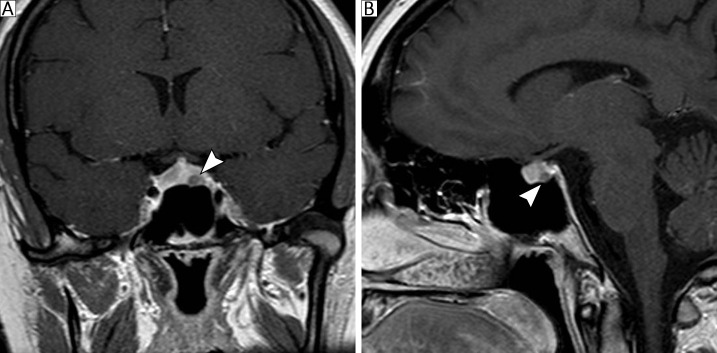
(A, B) Presurgical coronal and sagittal pituitary MR images showing a solid tumor lesion (4 mm) on the left side of the pituitary gland that was hypointense on T2-weighted images (white arrows). The lesion does not compress the pituitary stalk or the neurohypophysis.
